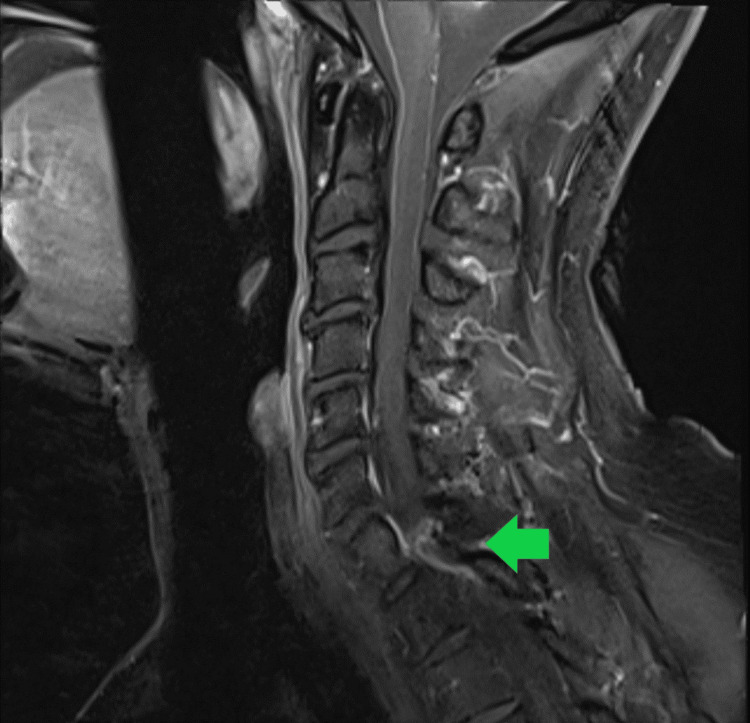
Contrast-enhanced sagittal MRI demonstrating the cyst.
